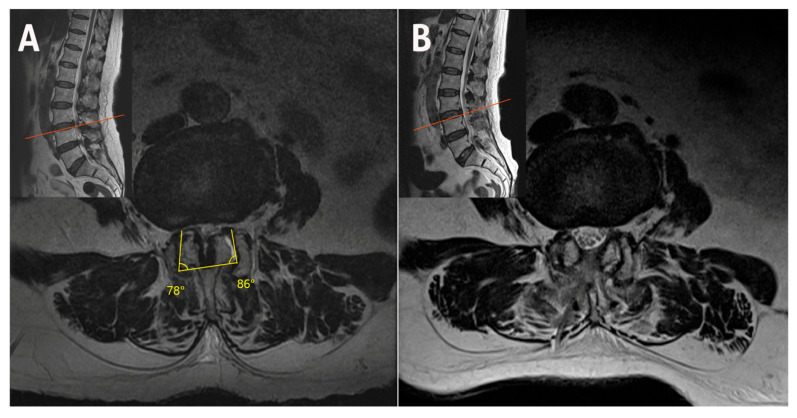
(A) Facet angles were measured as the angle between a line connecting the two ends of the facet joint surface and a reference line along the posterior margin. The average value (82.0 degrees in this case) was utilized when the facet angles differed (right side vs. left side). (B) Postoperative MRI after bilateral–contralateral decompression at L3–L4 showing preserved bilateral facet bases in vertically oriented joints. MRI, magnetic resonance imaging.
